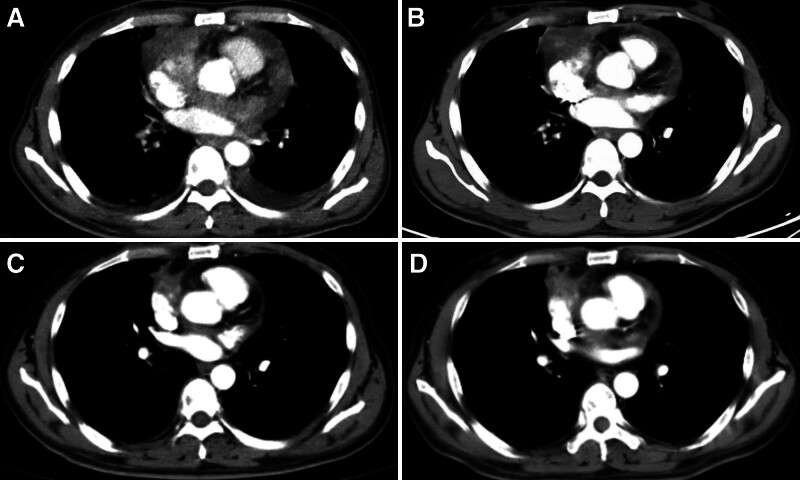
CT of PCA. (A) Before resection of the heart tumor (about 3.5 cm × 3.2 cm) on May 18, 2021. (B) Recurrent lesions after operation on March 31, 2021. (C) After 2 cycles of anlotinib on August 27, 2021, PR was evaluated. (D) After 4 cycles of anlotinib on October 24, 2021, PD was evaluated. CT = computed tomography, PCA = primary cardiac angiosarcoma, PD = progressive disease, PR = partial response.
